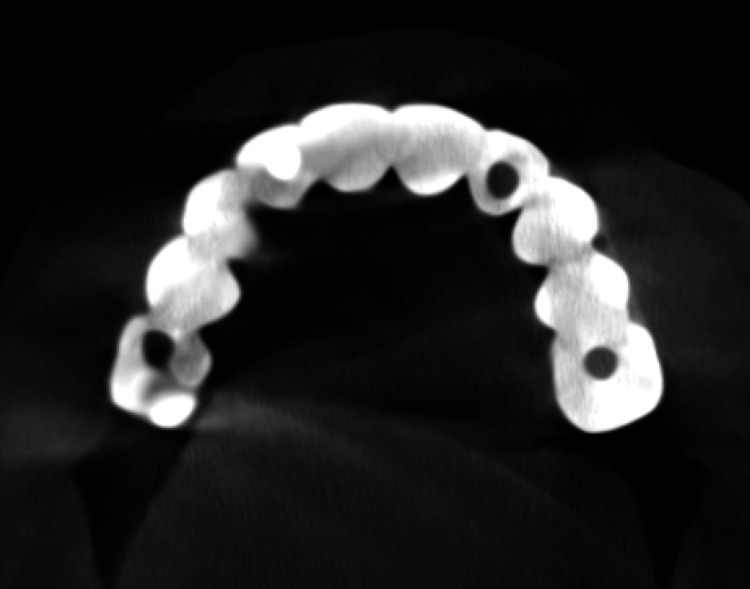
Occlusal view of the radiographic image of the prosthesis at delivery for evaluation of OnX implant-supported prosthesis.
